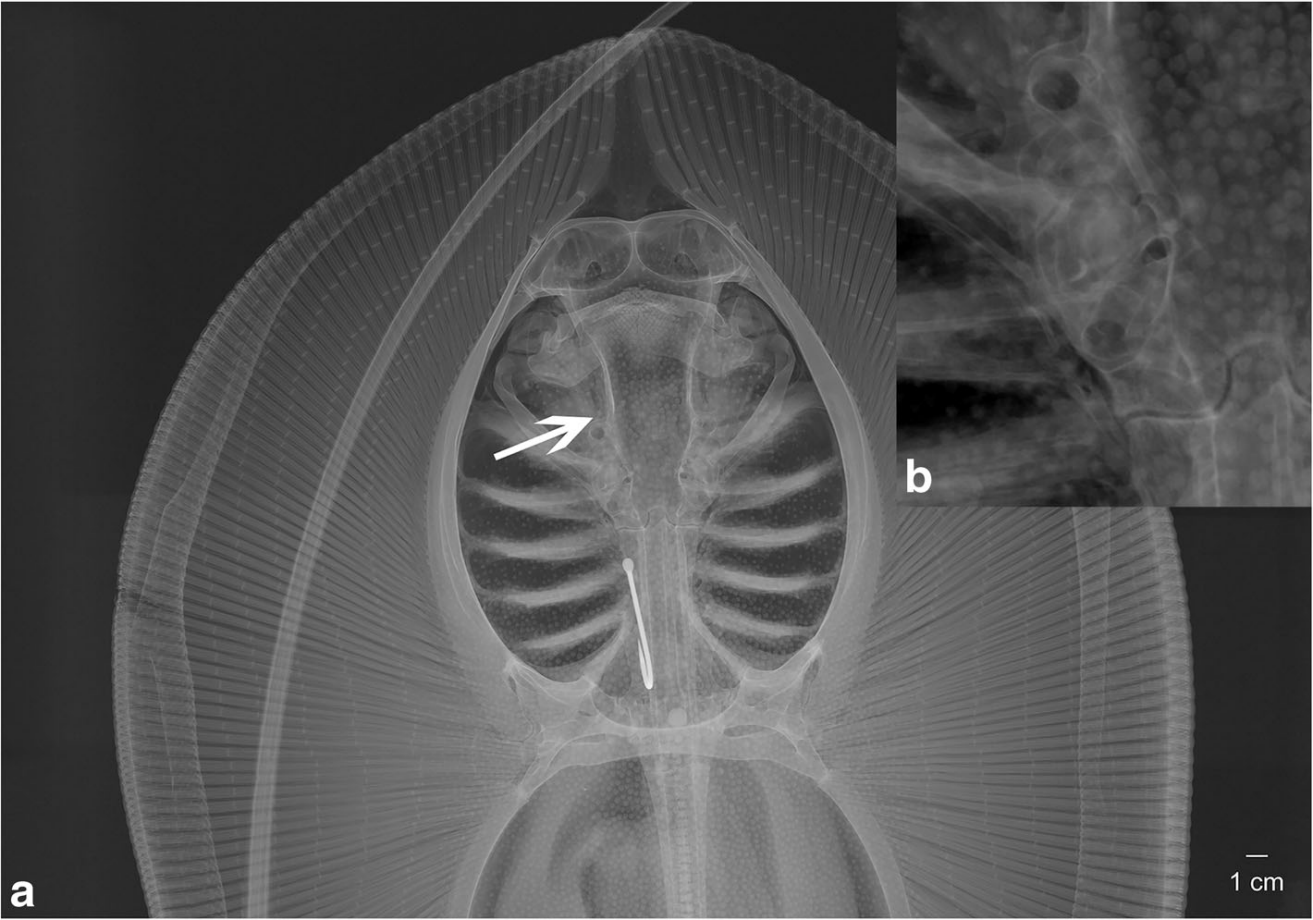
Radiograph of a female *Himantura signifer* (ZSM 30351) with the otolith structure (a, arrow). b) shows a close-up of the inner ear region
